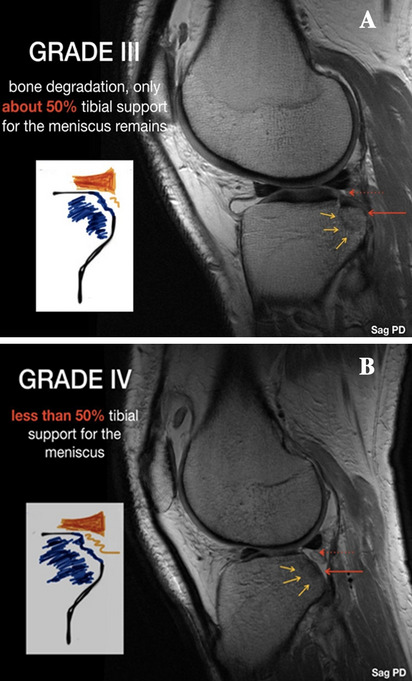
Typical impression fracture of the posterolateral zone of the tibial plateau according to Smigielski classification, seen in the sagittal plane MRI sequence of the right knee; A Grade III fracture with around 50% of remaining osseous support.; B Grade IV fracture with less than 50% of remaining osseous support
